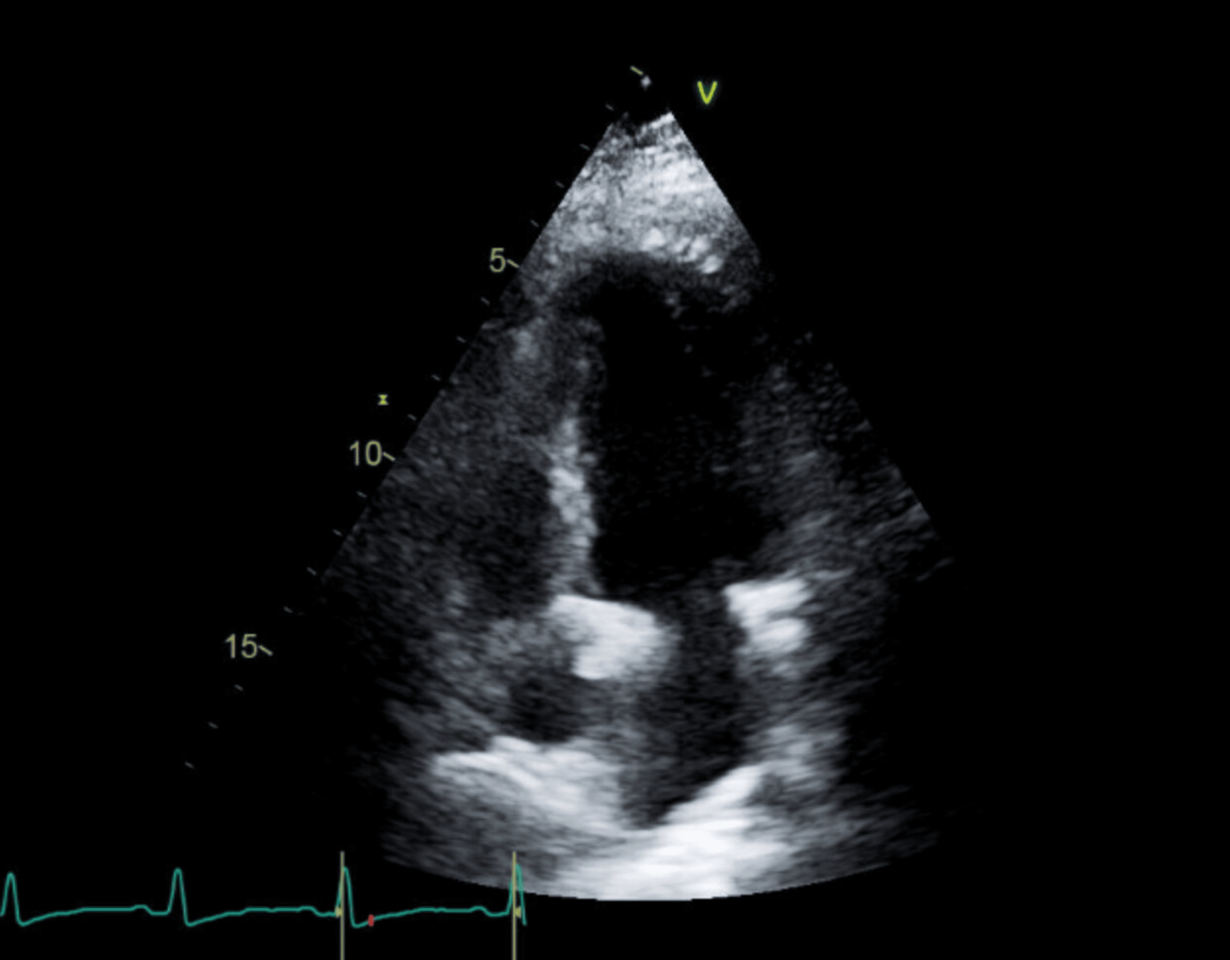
Apical view echocardiogram showing mild mitral valve leaflet thickening and tricuspid regurgitation.
